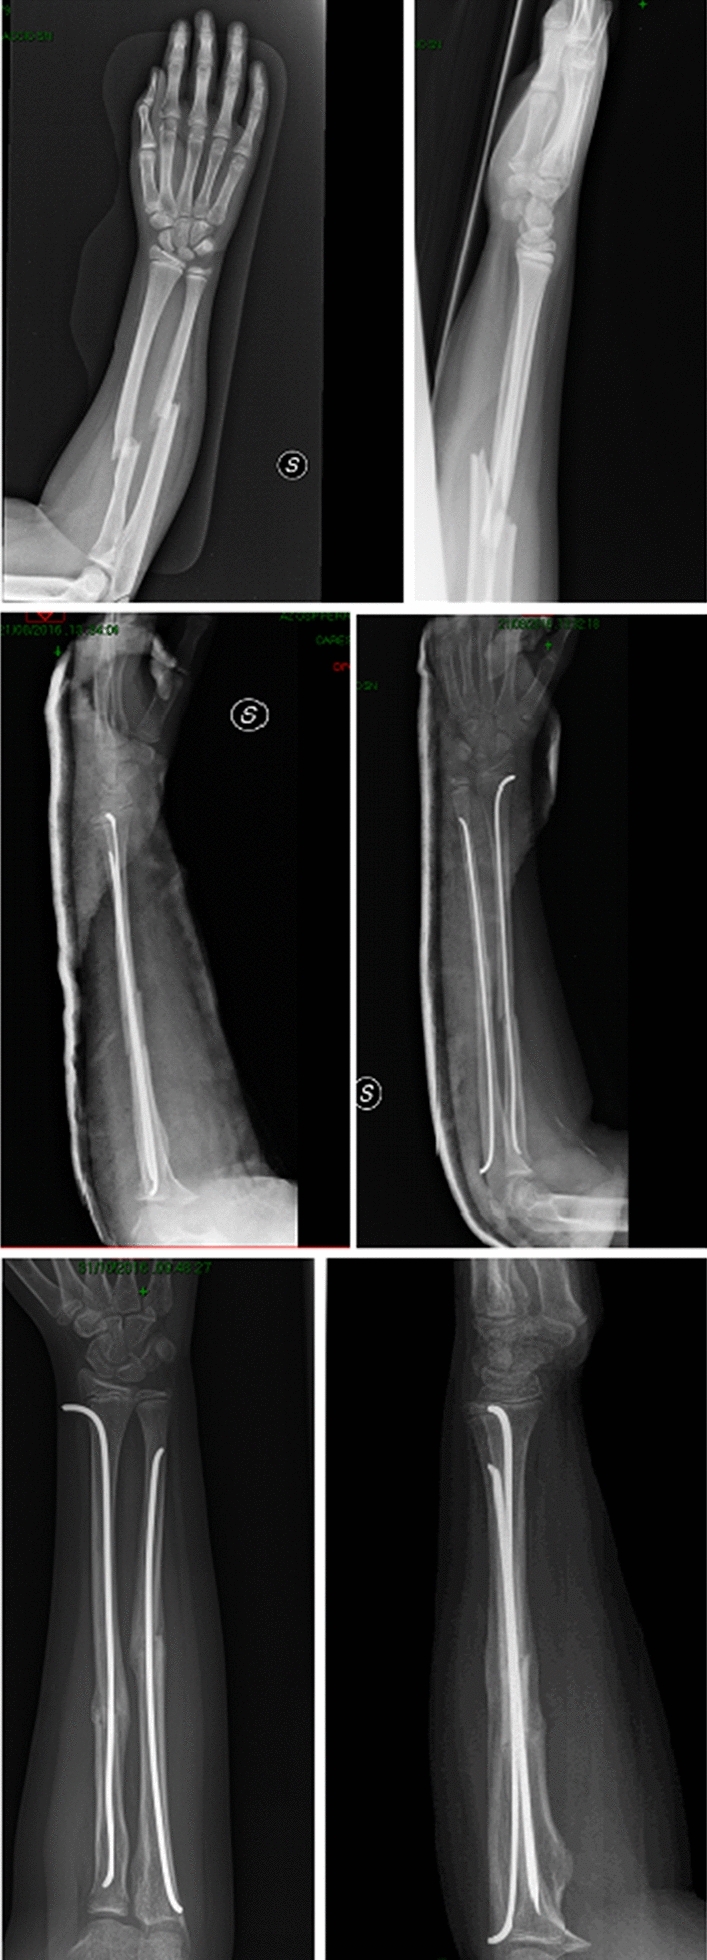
AP and lateral radiographic projection of both-bone forearm fractures treated with double elastic intramedullary nails technique
